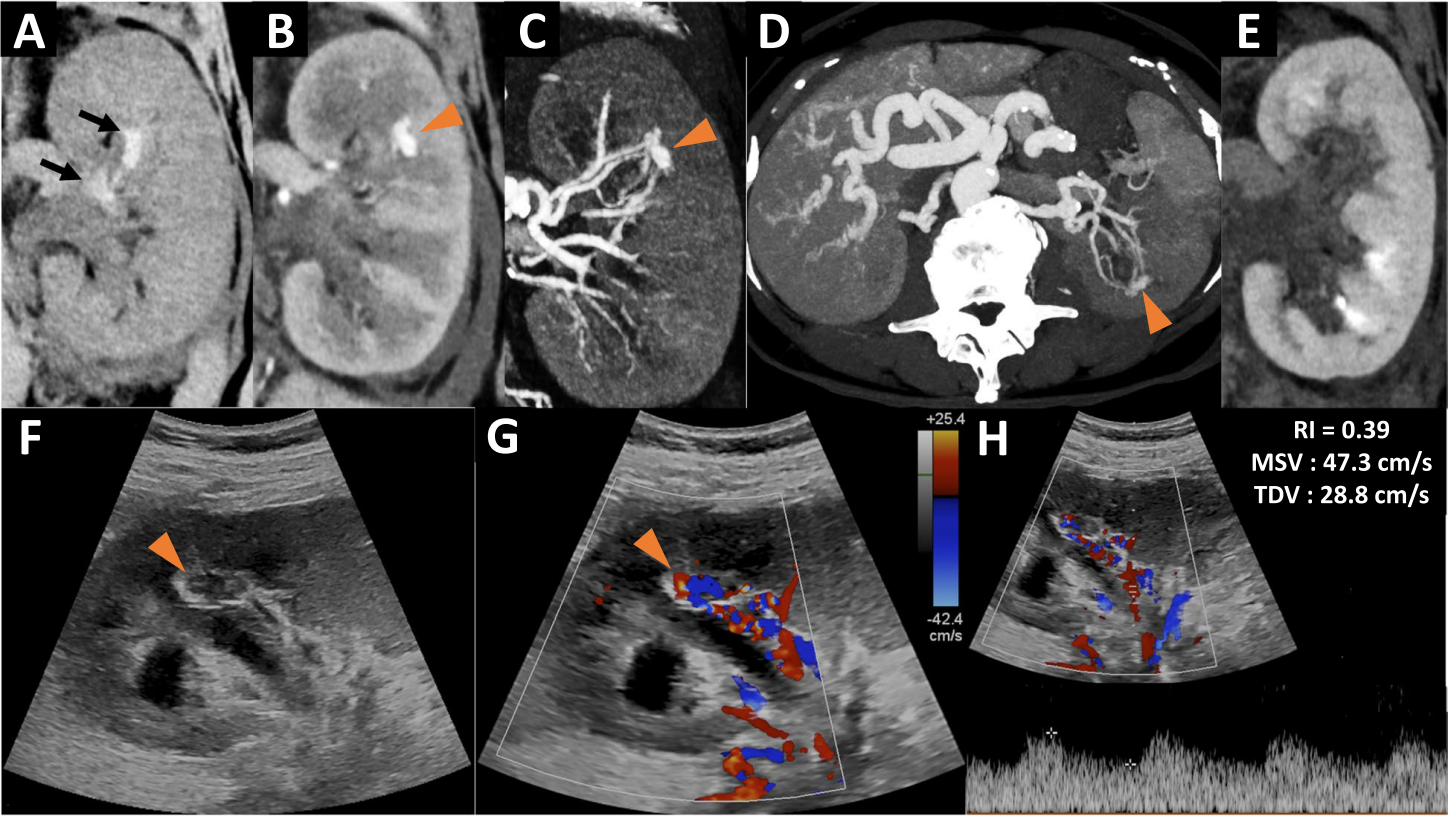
Computed tomography, ultrasound and doppler of left renal AVM (A) Unenhanced CT in coronal reconstruction showing spontaneous hyperdensities in the superior calyx and renal pelvis of the left kidney, corresponding to clotting (black arrows), with dilatation of the left upper urinary tract B, C & D Contrast-enhanced CT at the cortical phase in coronal (B), coronal MIP 15mm and axial MIP 20mm showing the left renal AVM (orange arrowhead) with several arterial feeders. Axial MIP reconstruction shows the liver involvement of HHT with hepatic artery > 6mm and distal subscapular telangiectases E Contrast–enhanced CT at the excretory phase showing a delay in urinary excretion due to blood clots, obstructing the left upper urinary tract F & G—B mode ultrasound (F) and doppler (G) showing left renal AVM as an hypoechoic, well-marginated lesion, close to the sinus (orange arrowhead). Arterial feeders are seen on doppler examination H – Pulsed doppler in an arterial feeder of the AVM showing reduced resistance index (IR = 0,39) with increased tele-diastolic velocity (TDV≈29cm/sec)
